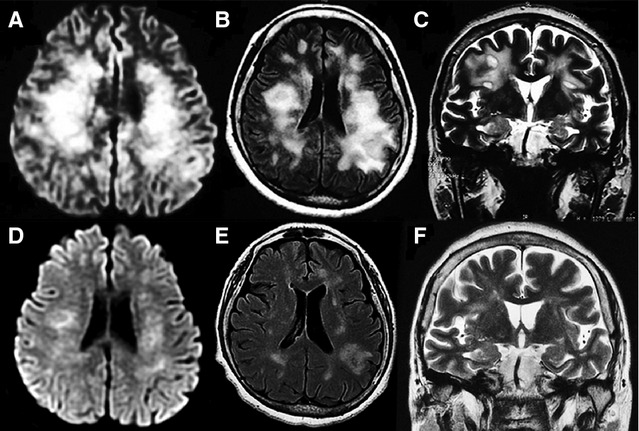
(A–C) MRI on diffusion, Fast-FLAIR, and T2-weighted sequences show extensive demyelinating areas involving subcortical white matter (bilateral centrum semi oval). (D–F) Control MRI performed 4 months after initial symptoms shows ostensible improvement of lesions and secondary subcortical atrophy.
